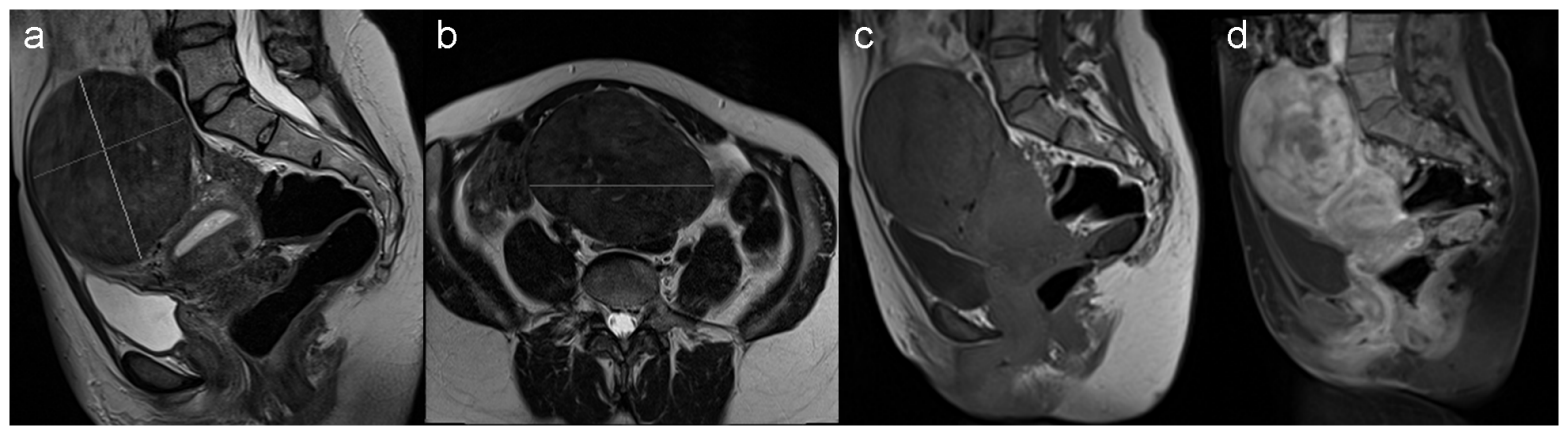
Sagittal (a) and axial-oblique (b) T2-weighted images showing dimensions of a large fibroid. (c) Magnetization transfer-weighted and (d) dynamic contrast-enhanced MRI (DCE-MRI) image (acquired one minute after injection of contrast) of the same patient.
